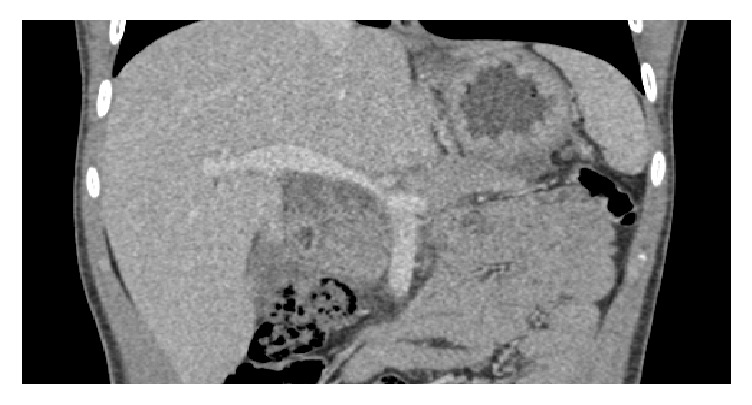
Abdominal CT showing a stage E pancreatitis.
